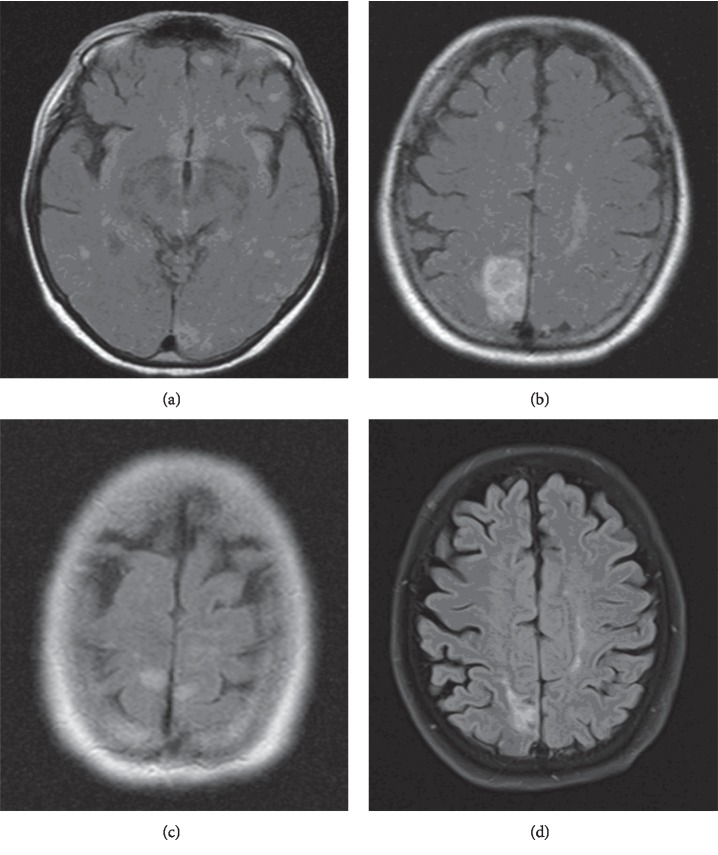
MRI brain showing acute ischemic regions. (a-b) Acute infarcts in the posterior circulation involving bilateral cerebellar hemispheres, medial bilateral occipital and parietal lobes, and the posterior watershed region at the vertex with small amount of hemorrhagic conversion within the right parietal parasagittal lesion. (c-d) MRI image of infarct in the medial occipital lobe and parietal lobes bilaterally.
